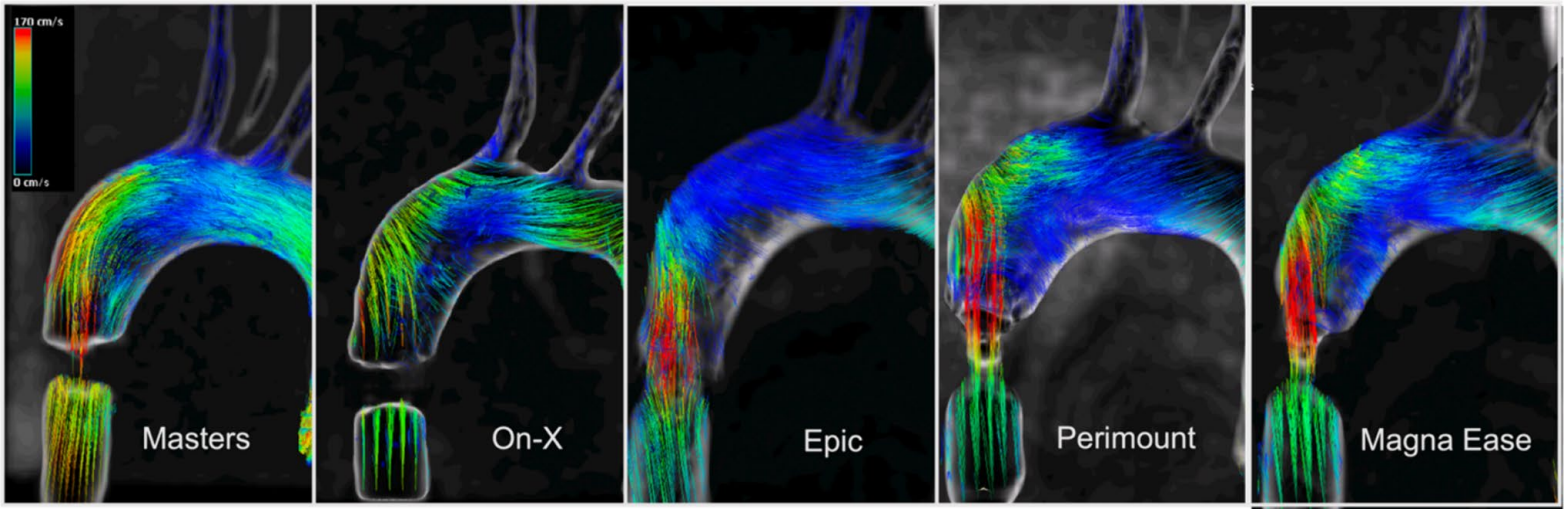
4D-MRI pathline visualization of the peak flow velocity in the aortic arch for all surgical aortic valves during peak systole; Mechanical valves showed artefacts around the valvular plane, leading to signal loss
